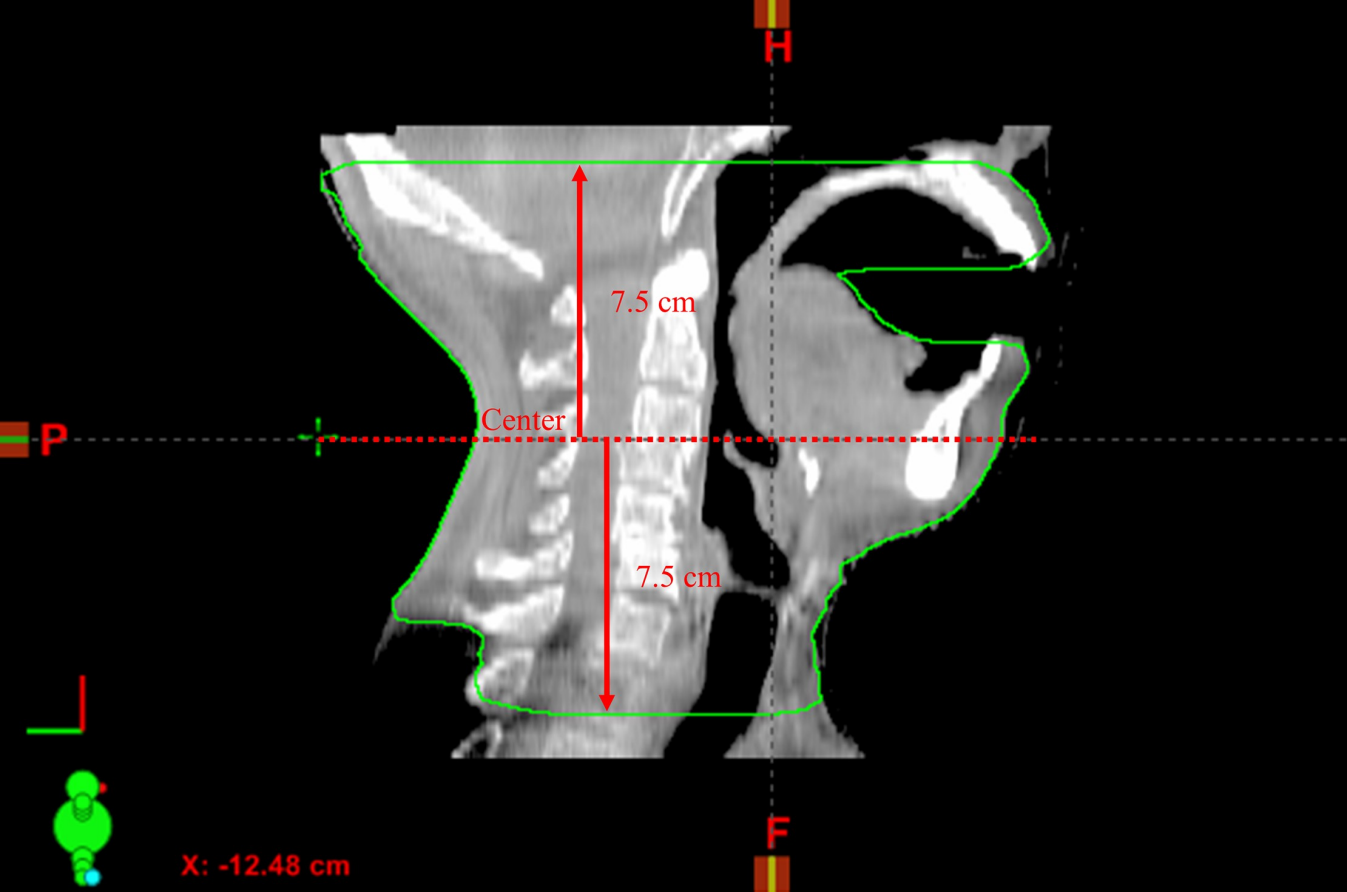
Body volume was measured using cone beam computed tomography. The measurement range was 7.5 cm in the superior and inferior directions from the centre of the CBCT.
